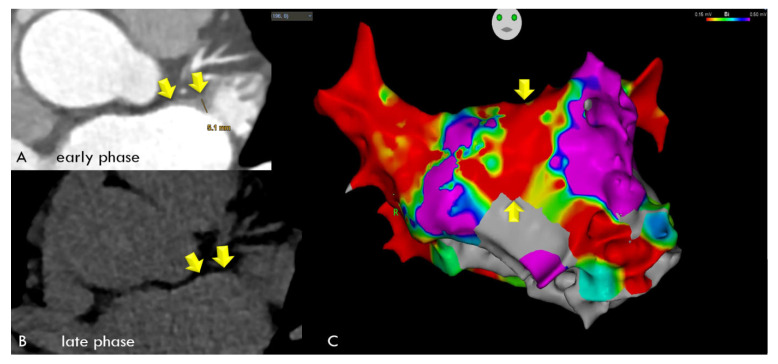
A 59-year-old male with a BMI of 27.8 kg/m^2^, and a LVEF of 68% with paroxysmal AFIB and severe left atrial wall thickening (LAWT) with a maximum of 5.1 mm during early-phase CT (Panel (A), arrows) and linear late enhancement during late-phase CT (Panel (B), arrows), indicating diffuse fibrosis, which was correlated with low-voltage areas along the entire anterior left atrial wall during electroanatomical mapping (EAM). (Panel (C)) All 20 spots were positive for low-voltage areas of <0.5 mV, shown as red areas (yellow arrows), with values ranging from 0.015 to 0.12 (mean: 0.024 mV). While LA ablation with pulmonary vein isolation was successful, the patient experienced recurrent AFIB after 3 months, and a second LA ablation was performed 7 months later.
